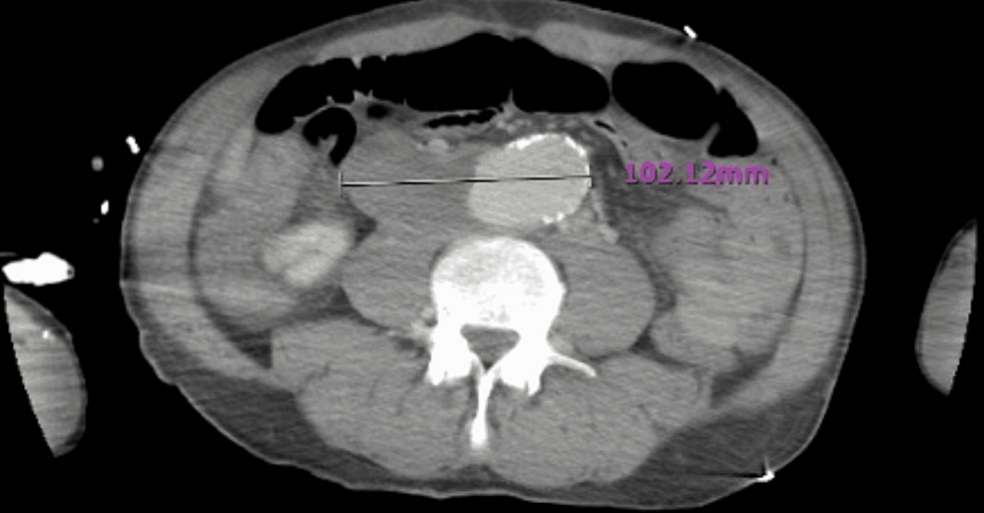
CT scan showing saccular aneurysm with thrombosed component
